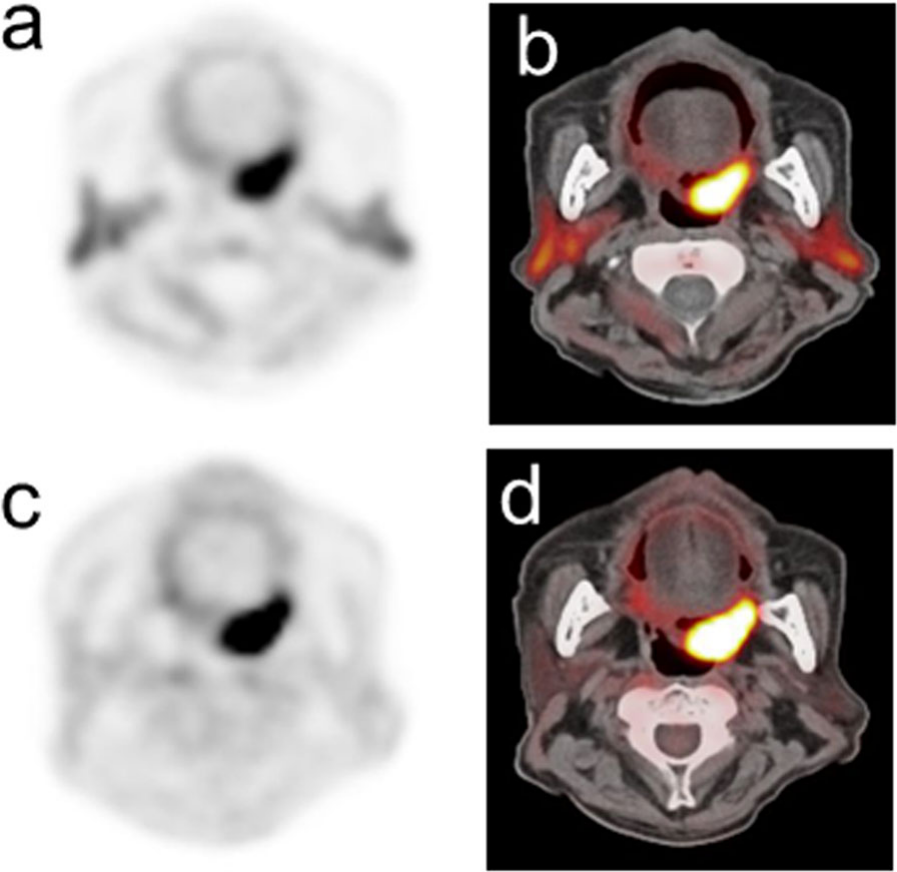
PET/CT images from a 66-year-old male diagnosed with p16-negative oropharyngeal squamous cell carcinoma in the soft palate. Transverse ^11^C-4DST PET image (a) and fused PET/CT image (b) show an increased uptake in the primary tumor (SUVmax = 8.00, homogeneity = 0.295, entropy = 2.050, SRE = 0.965, LRE = 1.140, LGZE = 0.00514, and HGZE = 260.00). Transverse ^18^F-FDG PET image (c) and fused PET/CT image (d) also show an increased uptake in the primary tumor (SUVmax = 14.57, homogeneity = 0.194, entropy = 1.970, SRE = 0.986, LRE = 1.060, LGZE = 0.00168, and HGZE = 843.90)
